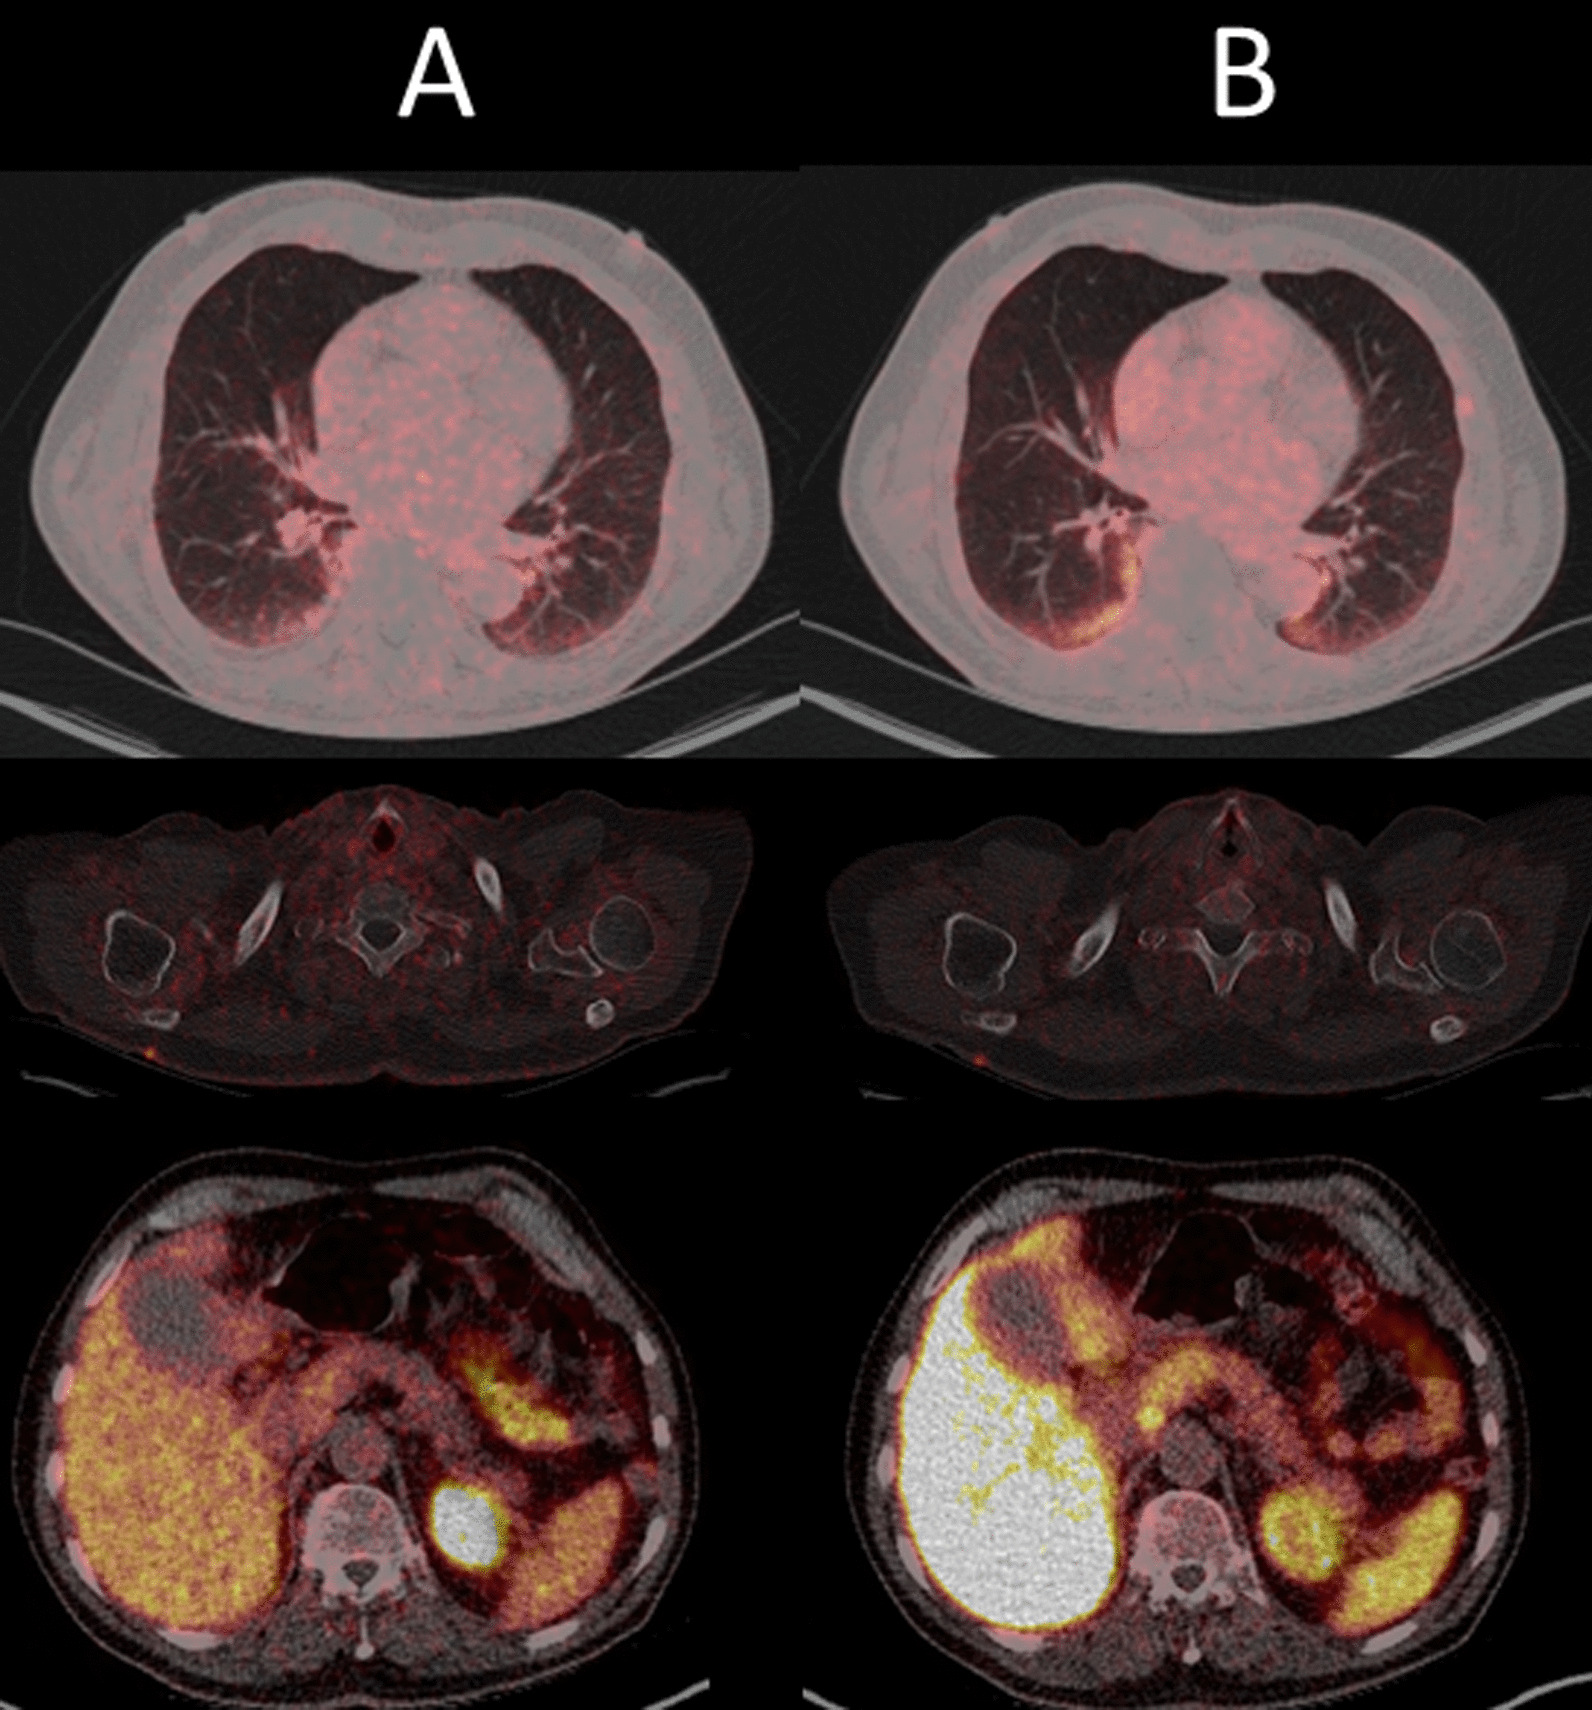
Axial fused PET/CT with concordantly presumable unspecific [^68^Ga]-Ga-PSMA-11 (A) and [^18^F]-F-PSMA-1007 PET/CT (B) uptake in bipulmonary infiltrations, as well as in a cutaneous lesion in the right dorsal thoracic wall, clinically evaluated as keloid scar
